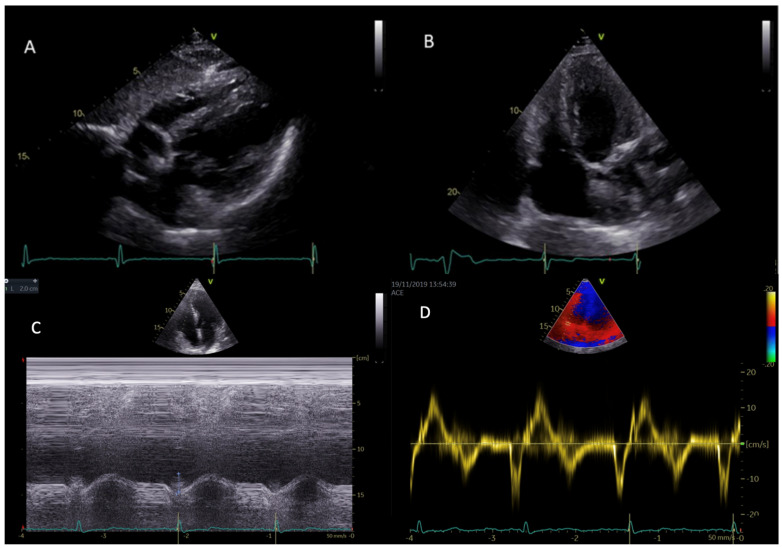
Right and left ventricular hypertrophy in subcostal view (A) and in modified apical four-chambers view (B) in two different patients with AFD. Parametric indexes of right ventricular function, namely TAPSE (tricuspid annular plane systolic excursion) (C) and RVS’ (TDI: tissue Doppler imaging-derived tricuspid lateral annular systolic velocity wave) (D) in apical four-chambers view.
